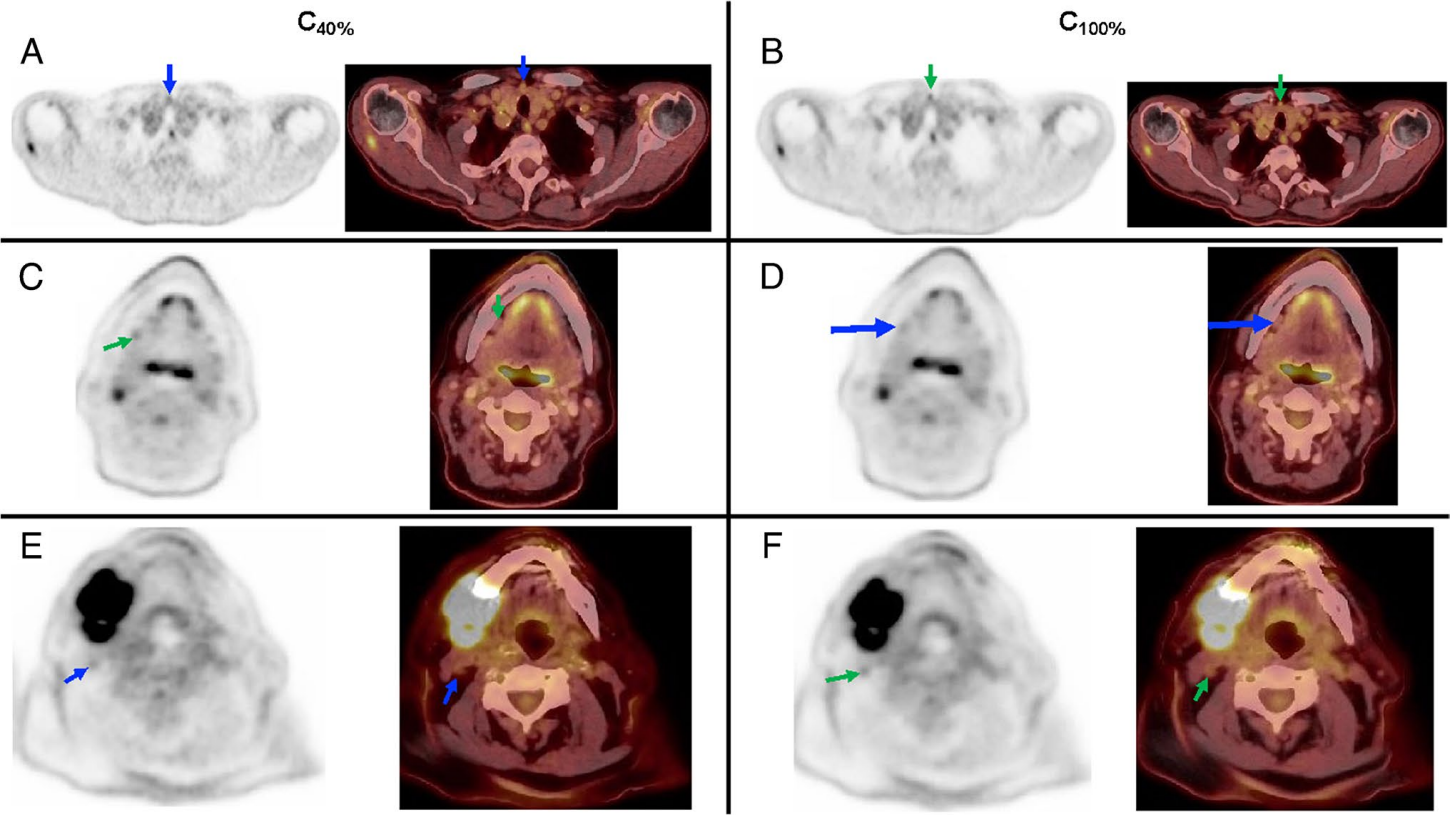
Example cases and positive lymph nodes (arrows) on the 4-min (C_40%_) and 10-min (C_100%_) acquisitions. A, B Patient with metastatic squamous cell oral carcinoma with a LN metastasis (level VI on the right), with better visualisation at C_100%_ acquisition (green arrow) rather than C_40%_ (blue arrow). C, D Patient with pharynx squamous cell carcinoma (SCC) shows multiple cervical LN metastases with a small submandibular lymph node with low FDG-uptake only at C_40%_ (green arrow), which was not appreciated in the C_100%_ (blue arrow). E, F A small lymph node adjacent to an enlarged node metastasis in the II level in a patient with oral cavity squamous cell carcinoma was detected only at C_100%_ (green arrow), with suboptimal visualisation at C_40%_ (blue arrow)
